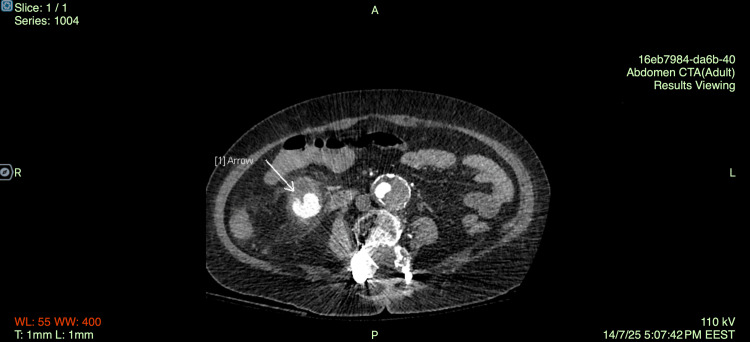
Axial CT angiography of the abdomen at the level of the infrarenal abdominal aorta demonstrates a fusiform aneurysmal dilatation with circumferential wall calcifications and probable mural thrombus.
